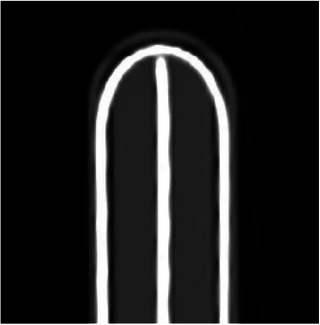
CT scan image of the HZ in coronal section. The highlighted lines are marker wires, which are placed in the U‐shaped channel and the center channel.
